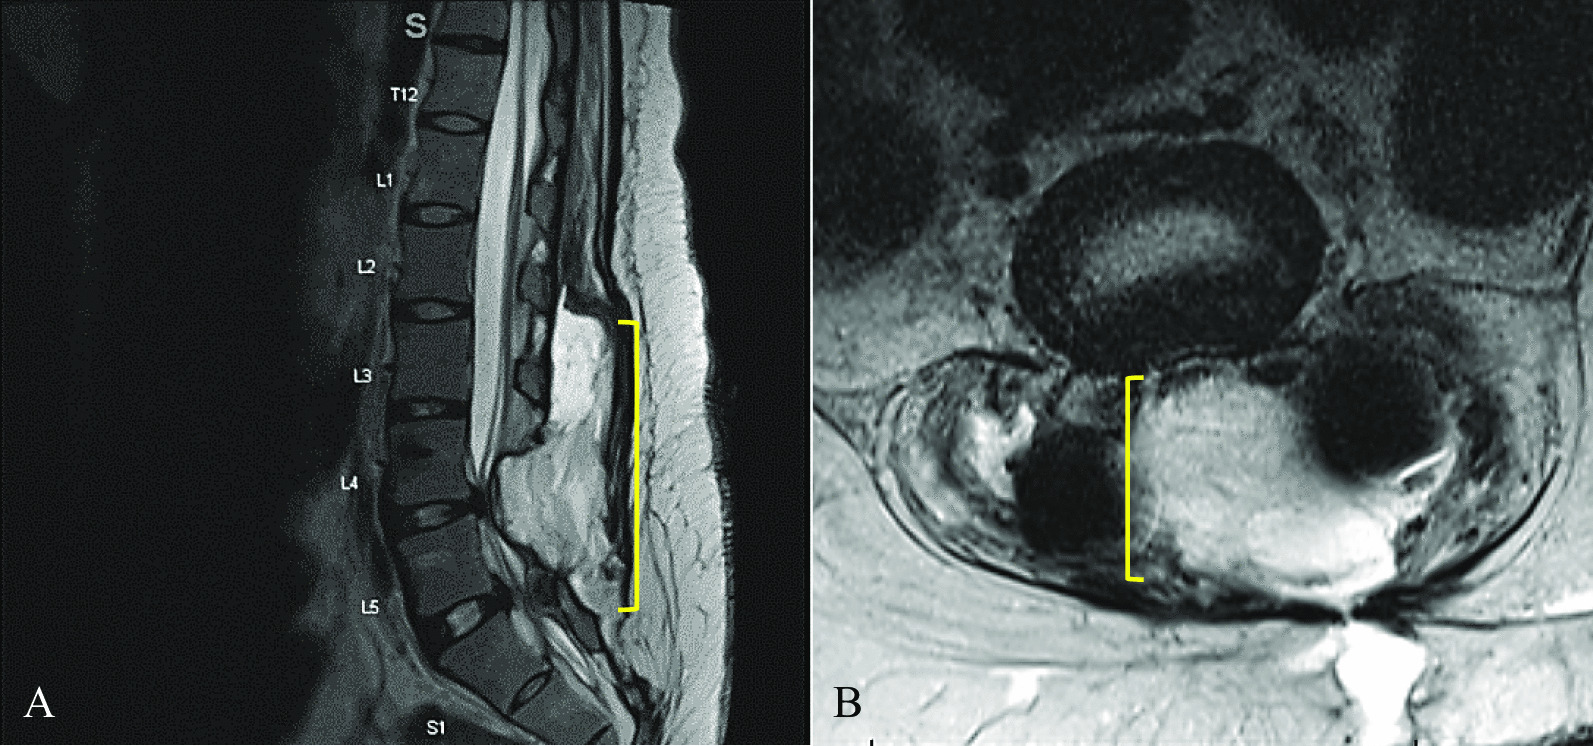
Postoperative images obtained 16 days after redo lumbar decompression and fusion surgery demonstrating surgical site infection. a Postoperative T2-weighted sagittal MRI showing surgical site fluid collection (bracket) compressing the thecal sac at L4–L5. b Postoperative T2-weighted axial MRI showing surgical site fluid collection (bracket) compressing the thecal sac at L4–L5
